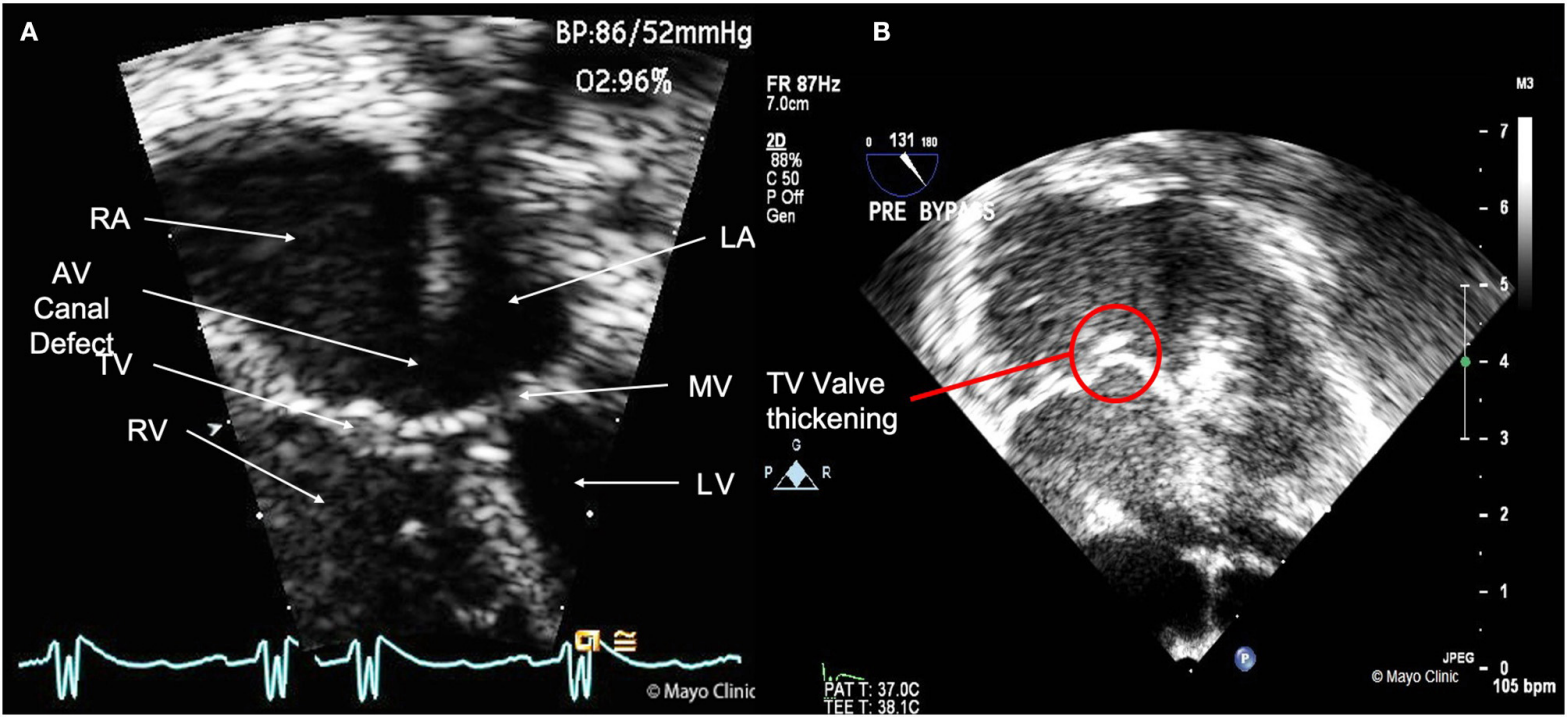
(A) Transthoracic echocardiography image showing the 4 cardiac chambers (RA, Right atrium; RV, Right ventricle; LA, left atrium; LV, Left ventricle), mitral valve (MV), tricuspid valve (TV), and the partial AV canal defect. (B) Intra-Op transesophageal echocardiography image, with view of the thickened TV.
